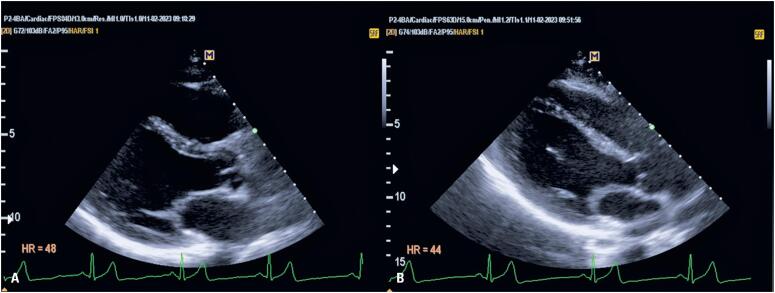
Comparison of parasternal long-axis images between the conventional position (A) and prone position (B)
